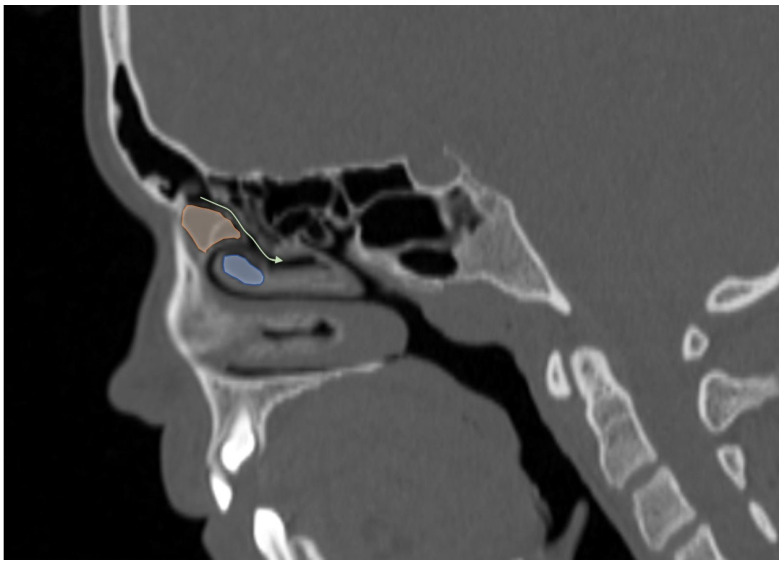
Sagittal reformatted CT image of a 14-year-old girl showing Agger Nasi cell (orange area) in front of the frontal recess draining into the middle meatus (curved arrow) and concha bullosa (blue area).
